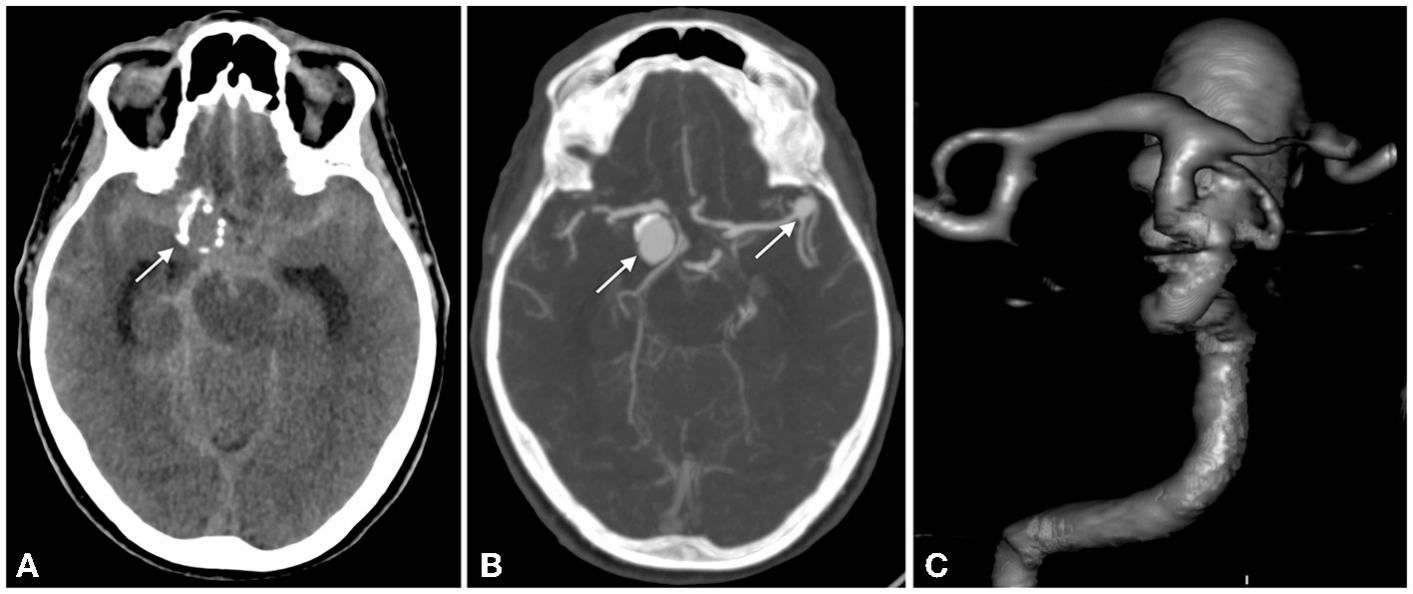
NCCT: subarachnoid hemorrhage in the basal and sylvian cistern >1 mm thick and in the 4^th^ ventricle; a round mass with peripheral calcification is seen (white arrow) (A); CTA and VRT display 2 aneurysms, one on the right supraclinoid ICA, the second on the left MCA bifurcation (white arrows) (B,C).
